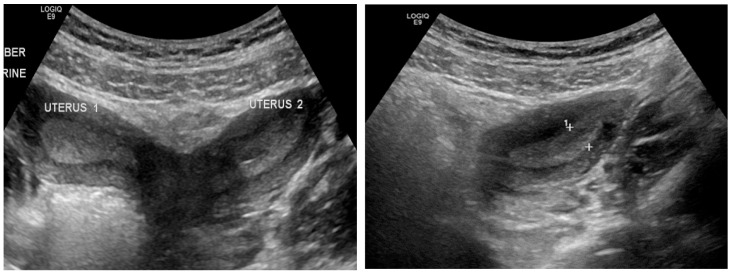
Postoperative follow-up transabdominal ultrasound in Case 2 demonstrating complete resolution of hematocolpos with restoration of normal vaginal anatomy following hemivaginal septal resection.
